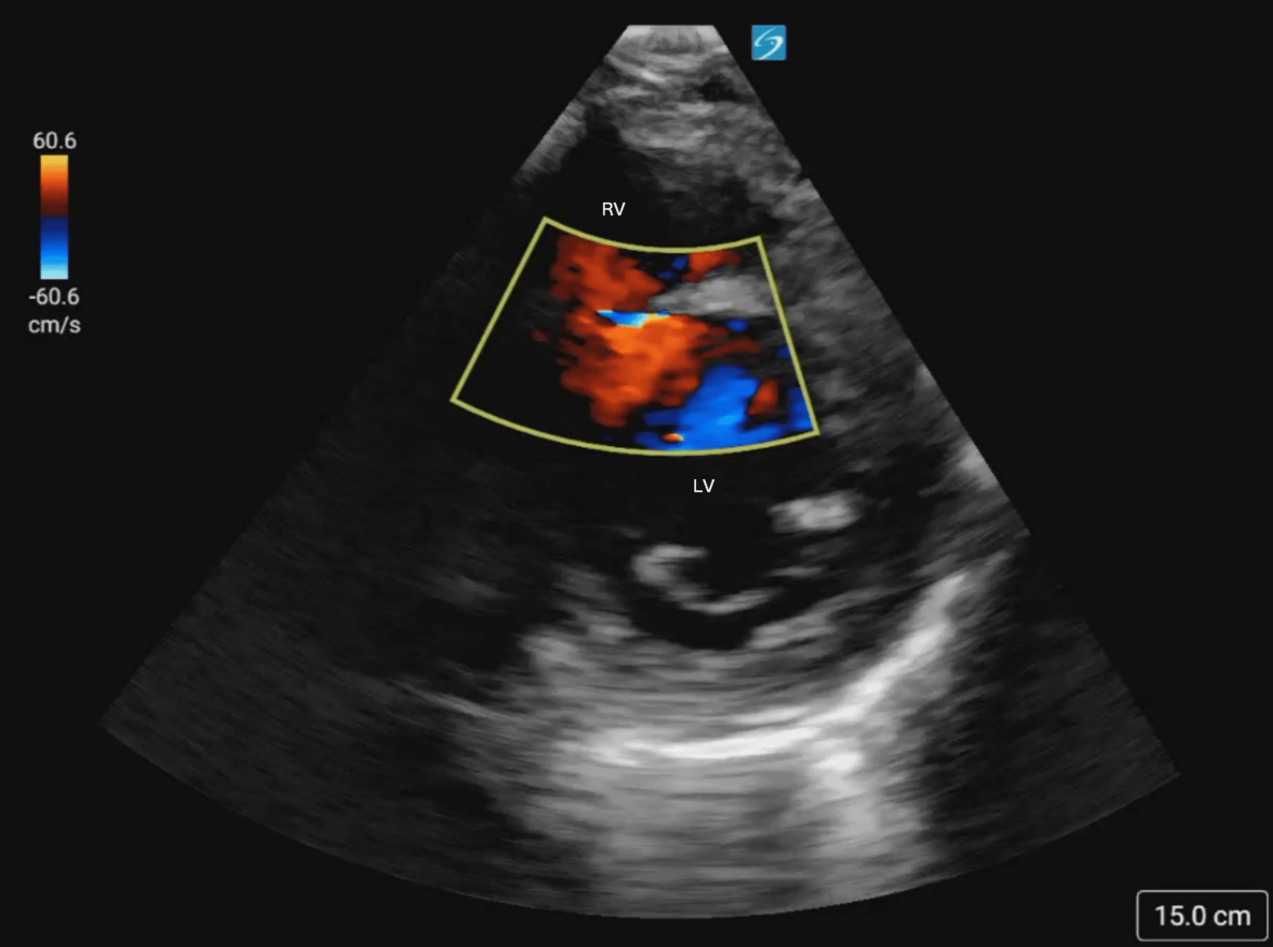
Parasternal short axis with color doppler view showing additional view with turbulent flow across septal wall
